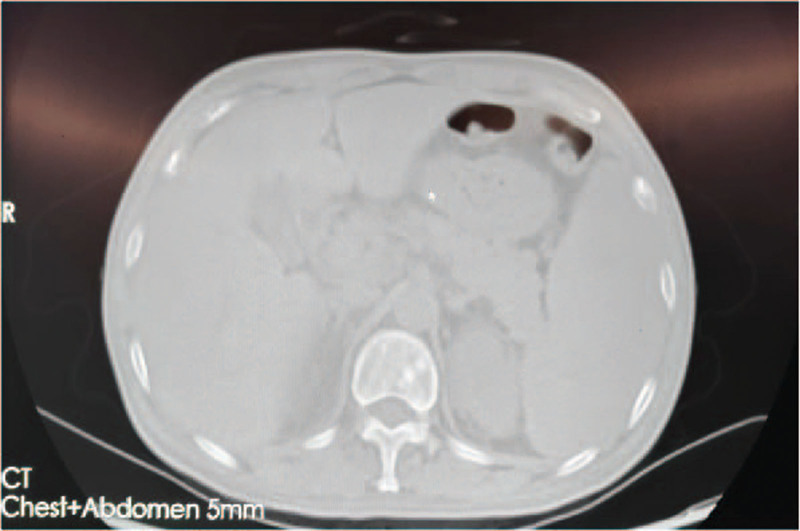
Multiple bilateral osteogenic changes in the enhanced CT.
